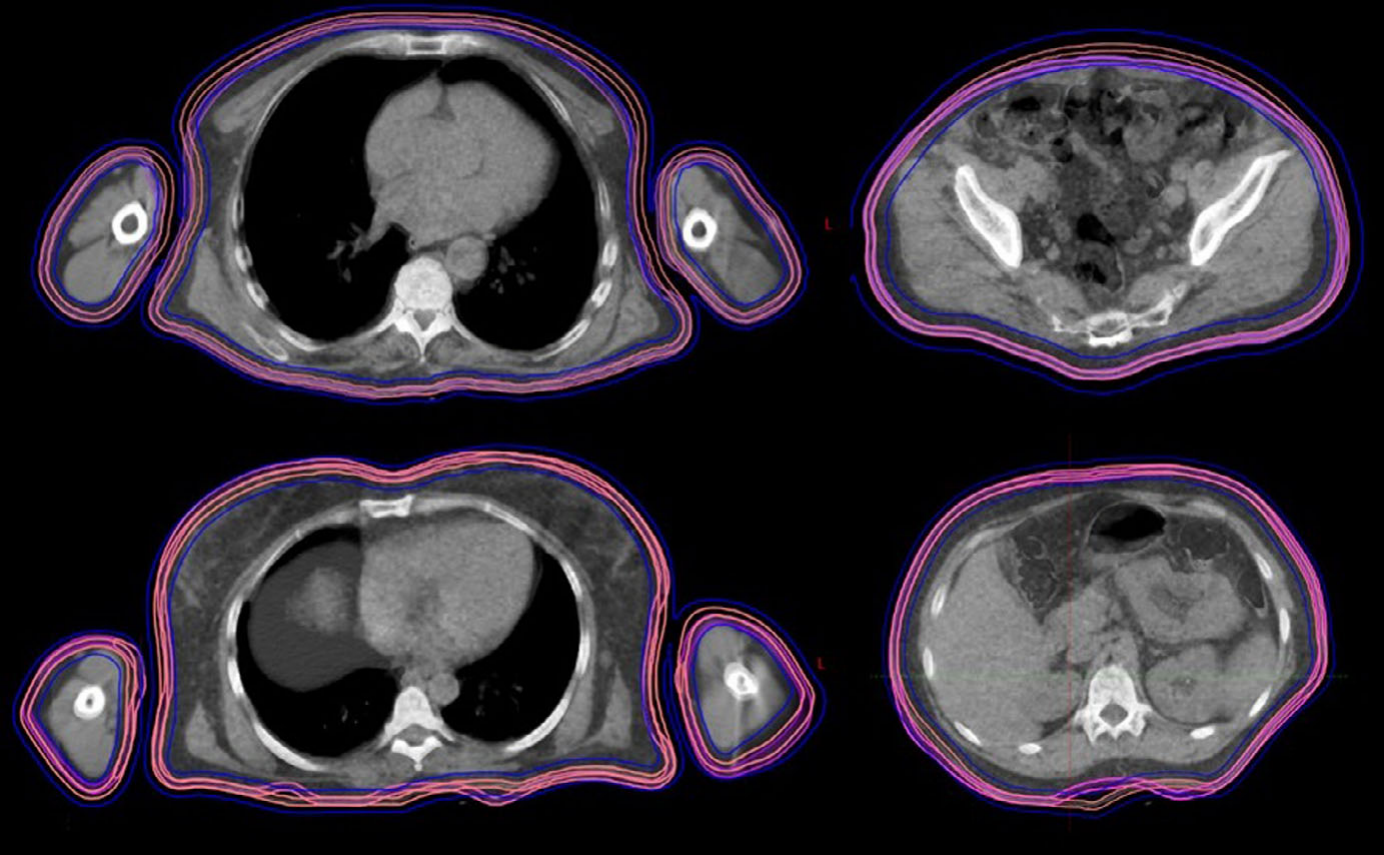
Daily megavoltage computed tomographic (MVCT) from the first fraction compared to planning computed tomography (CT) for patient 1 and 2. The CT and daily MVCT are overlayed with 50% transparency. Transversal slices for abdomen and thorax are displayed with original clinical target volume (CTV) (pink) with CTV for daily MVCT (magenta) and planning target volume (blue) from planning CT.
